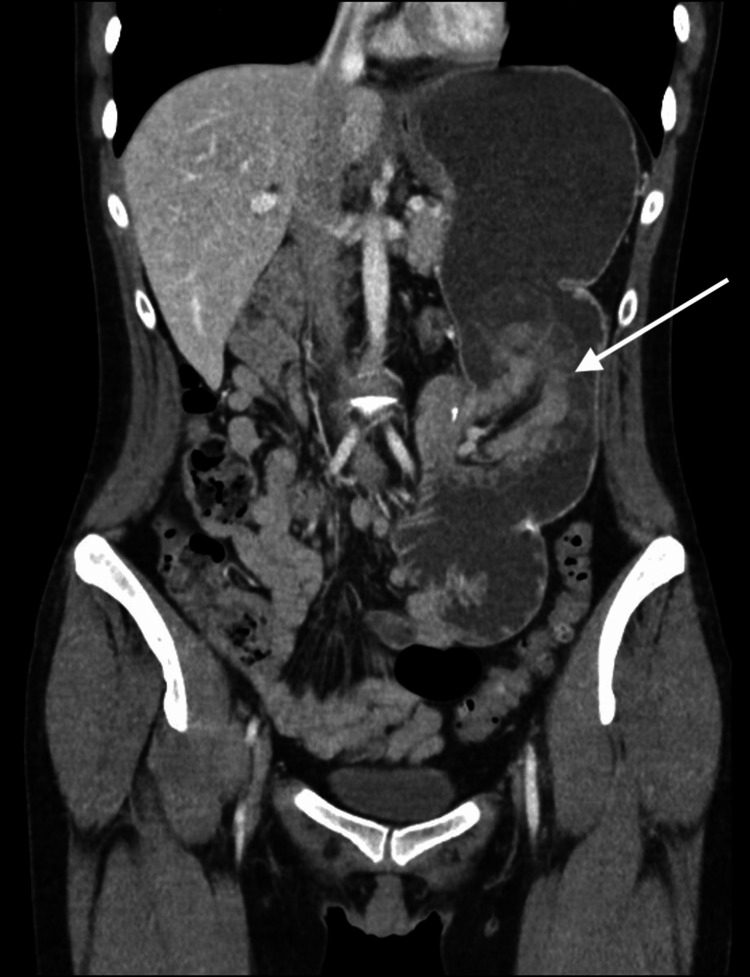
CT scan (coronal plane). Findings consistent with jejunal loop intussusception at the gastrojejunal anastomosis. Arrow: the examination reveals jejunal loop intussusception at the gastrojejunal anastomosis, resulting in marked dilation of the gastric chamber, without evidence of transmural ischemia of the intussuscepted loop.
